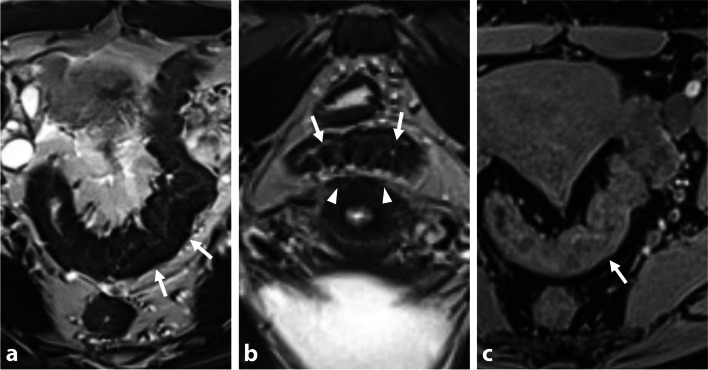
Normal sigmoid colon filled with feces in a 35-year-old woman with chronic pelvic pain. No medical history. a Axial and (b) coronal T2-W MR images show a T2-hypointense “pseudo”-thickening of the posterior sigmoid colon wall (arrows). Note the absence of retrocervical deep infiltrating endometriosis (arrowheads). c Axial T1-W fat-suppressed MR image shows the absence of hyperintense foci nor abnormality of the sigmoid colon wall (arrow), with a more or less fecal content. Laparoscopy showed a normal recto-sigmoid colon
